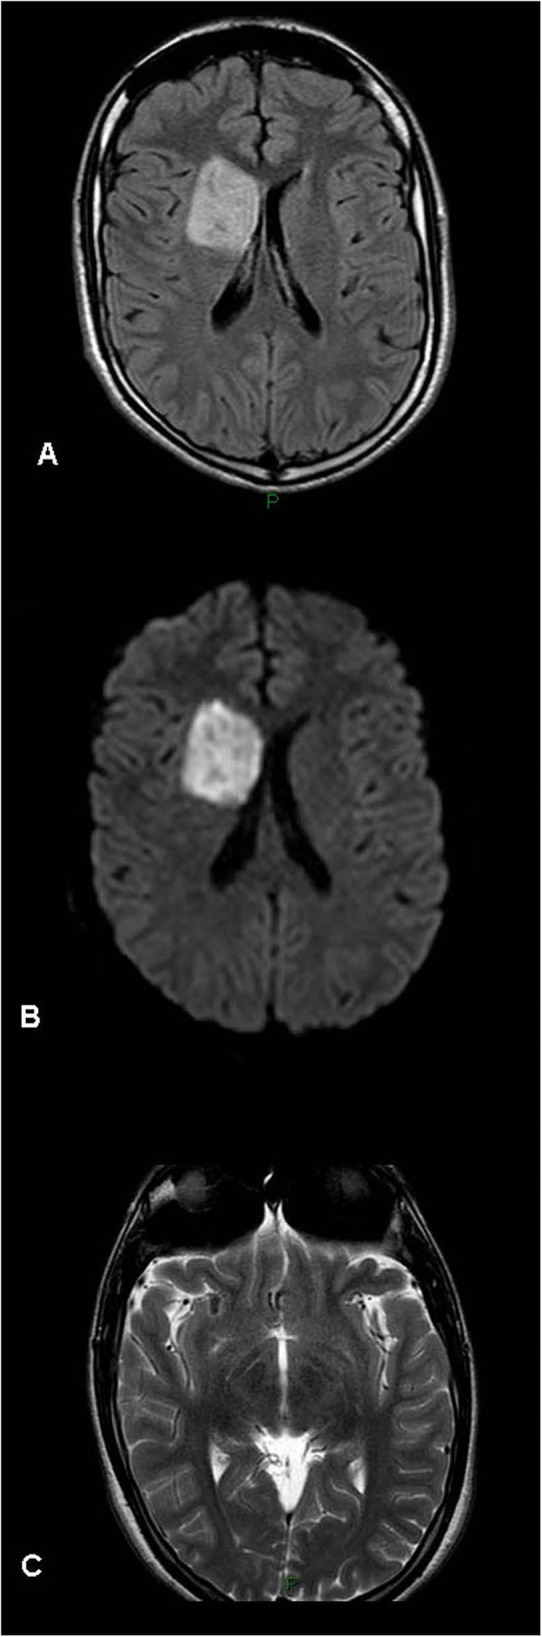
Acute right basal ganglia infarct – magnetic resonance (MR) findings (patient 7, Table 1). (A) FLAIR. (B) DWI. (C) T2 imaging. Right acute basal ganglia infarct with right M2 segment pseudoaneurysm visualized on the T2 weighted imaging, consistent with the patient history of Marfan disease.
